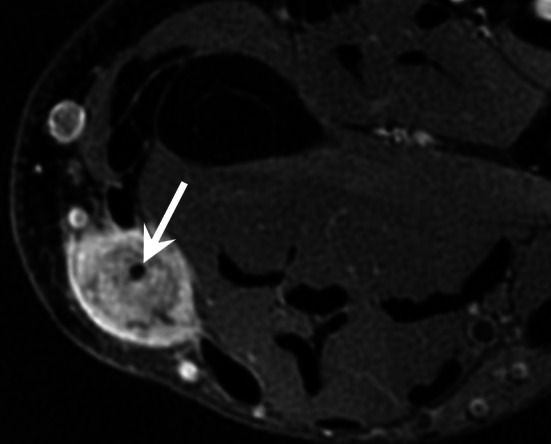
Epithelioid haemangioma of the RA. Axial T2-weighted MR image showing a hypervascular tumour arising from the arterial wall of the RA associated with narrowing of the vessel lumen (arrow)
